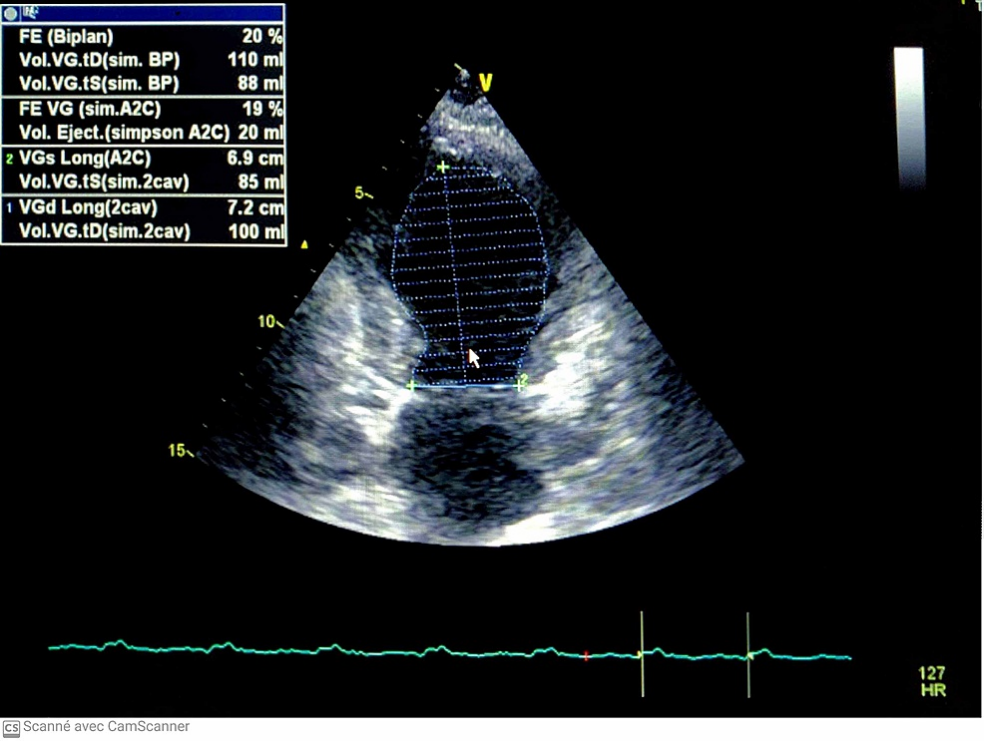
The echocardiography showed a dilated cardiomyopathy with severe EF 20%. EF, ejection fraction
